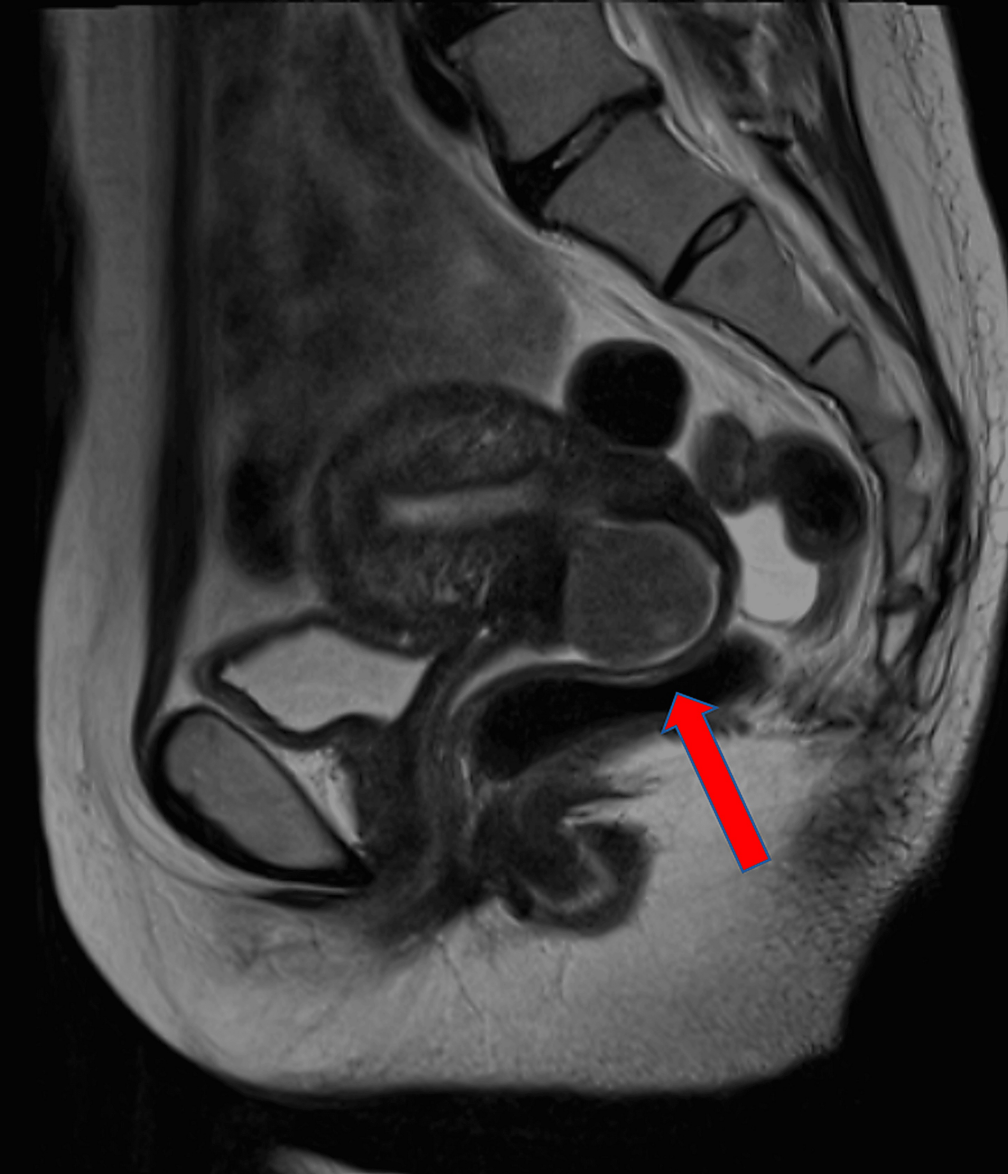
Sagittal pelvic MRI of a prolapsed submucosal myoma Sagittal pelvic MRI showing a pedunculated submucosal myoma prolapsing through the cervical canal. The red arrow indicates the protruding portion of the myoma beyond the external cervical os. MRI: magnetic resonance imaging
